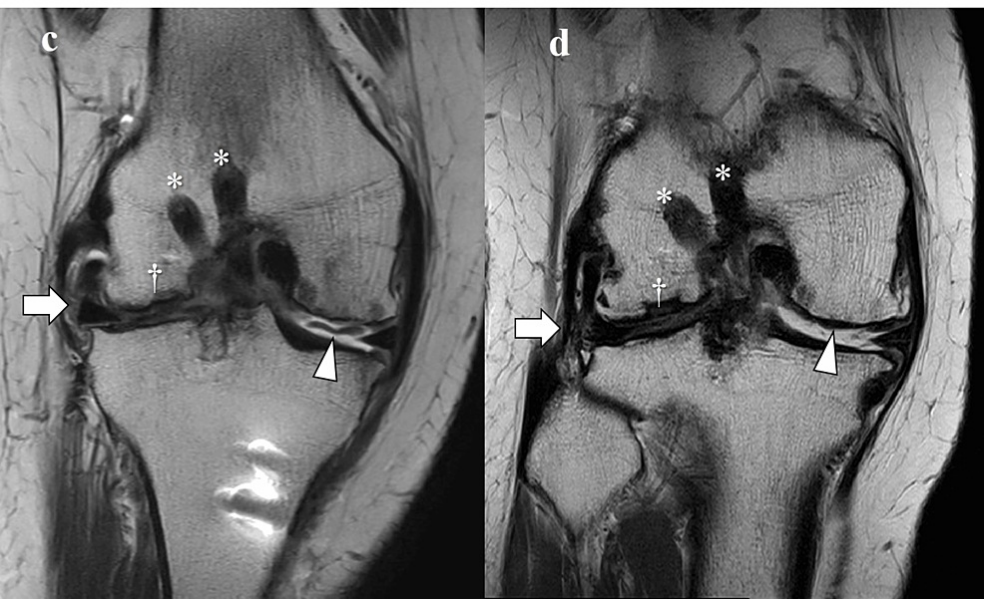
Coronal views of MRI (Case #4) - image 2 c. T2-weighted image (TR6000, TE 8.68, FOV:140) 12 months postoperatively. d. Proton density-weighted image (TR2400, TE 55.00, FOV:160) 24 months postoperatively *: Reconstructed anterior cruciate ligaments. †: Osteochondral autograft. White arrow: transplanted meniscal allograft. Arrowheads: autologous chondrocyte transplantation MRI: magnetic resonance imaging
